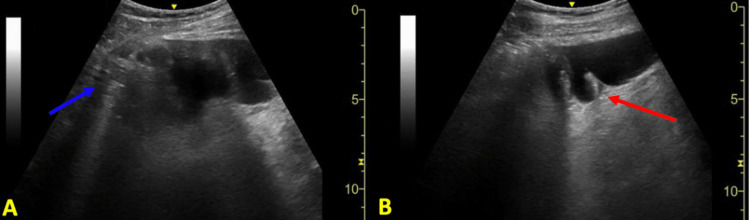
Sonographic image of the left lower quadrant Bedside point-of-care ultrasound (POCUS) image utilizing a low-frequency curvilinear probe at the left lower quadrant showing proximal (A) and distal (B) views of the distal descending colon. There is colonic dilation measuring maximally at about 5 cm. There are thickened plicae circulares measuring approximately 3 mm (red arrows) and abdominal A lines (blue arrow).
